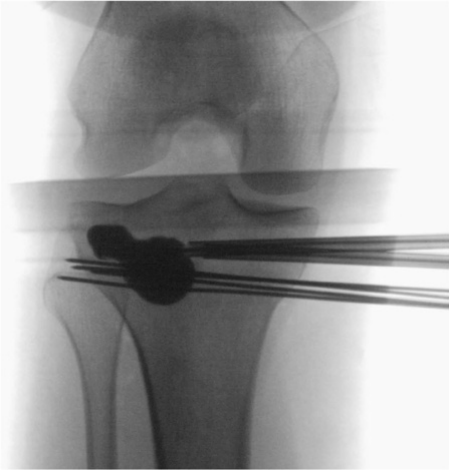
Intraoperative anteroposterior X-ray view of a minimally invasive, balloon-assisted reduction and cement-augmented internal fixation of a tibial plateau. Since the first balloon evaded distally, four Kirschner wires were used to buttress two balloons to cause the elevation of the impression and the final, successful reduction
